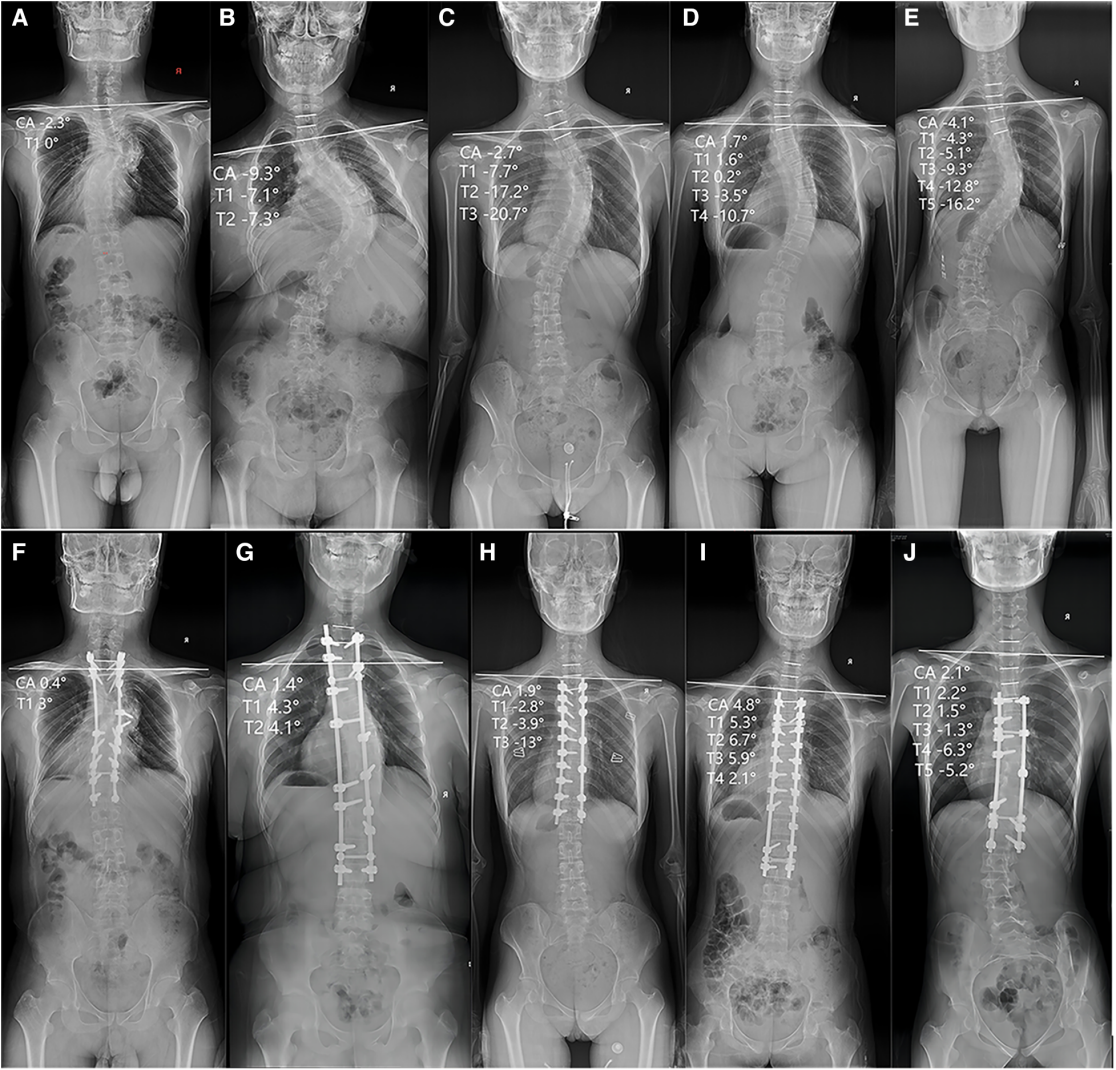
Representative scoliosis cases in the retrospective study and includes the AP radiograph of the entire spine preoperatively and the AP radiograph of the entire spine postoperatively. The (A) and (F) represent the preoperative and postoperative entire spine radiograph of patients with UIV located in T1, respectively. The (B) and (G) represent the preoperative and postoperative entire spine radiograph of patients with UIV located in T2, respectively, and the same applies to (C,H), (D,I) and (E,J).
